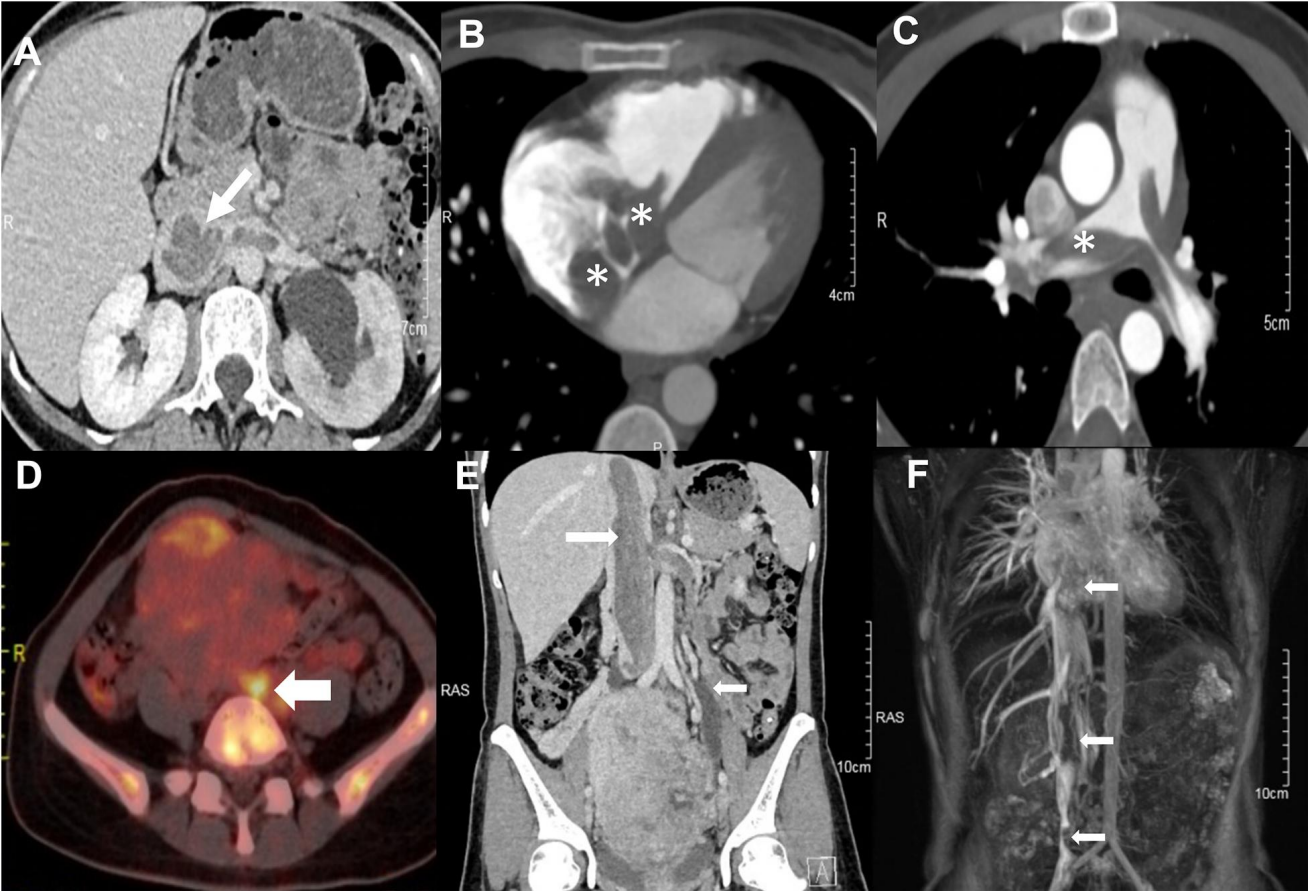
Preoperative imaging findings. (A) Axial view showing the presence of tumor thrombus in the left renal artery and extending into the inferior vena cava. (B) Axial view revealing the tumor thrombus extending from the right atrium through the tricuspid valve into the right ventricle. (C) Axial view demonstrating tumor thrombus within the main pulmonary artery as well as the left and right pulmonary arteries. (D) Fused PET/CT image showing the hypermetabolic tumor thrombus in the inferior vena cava and the left ovarian vein. (E) Coronal view showing the extensive tumor thrombus in the inferior vena cava (large arrow) and the left ovarian vein (small arrows). (F) Coronal view from magnetic resonance venography (MRV) confirming the extensive filling defects in the inferior vena cava and right ventricle (arrows).
